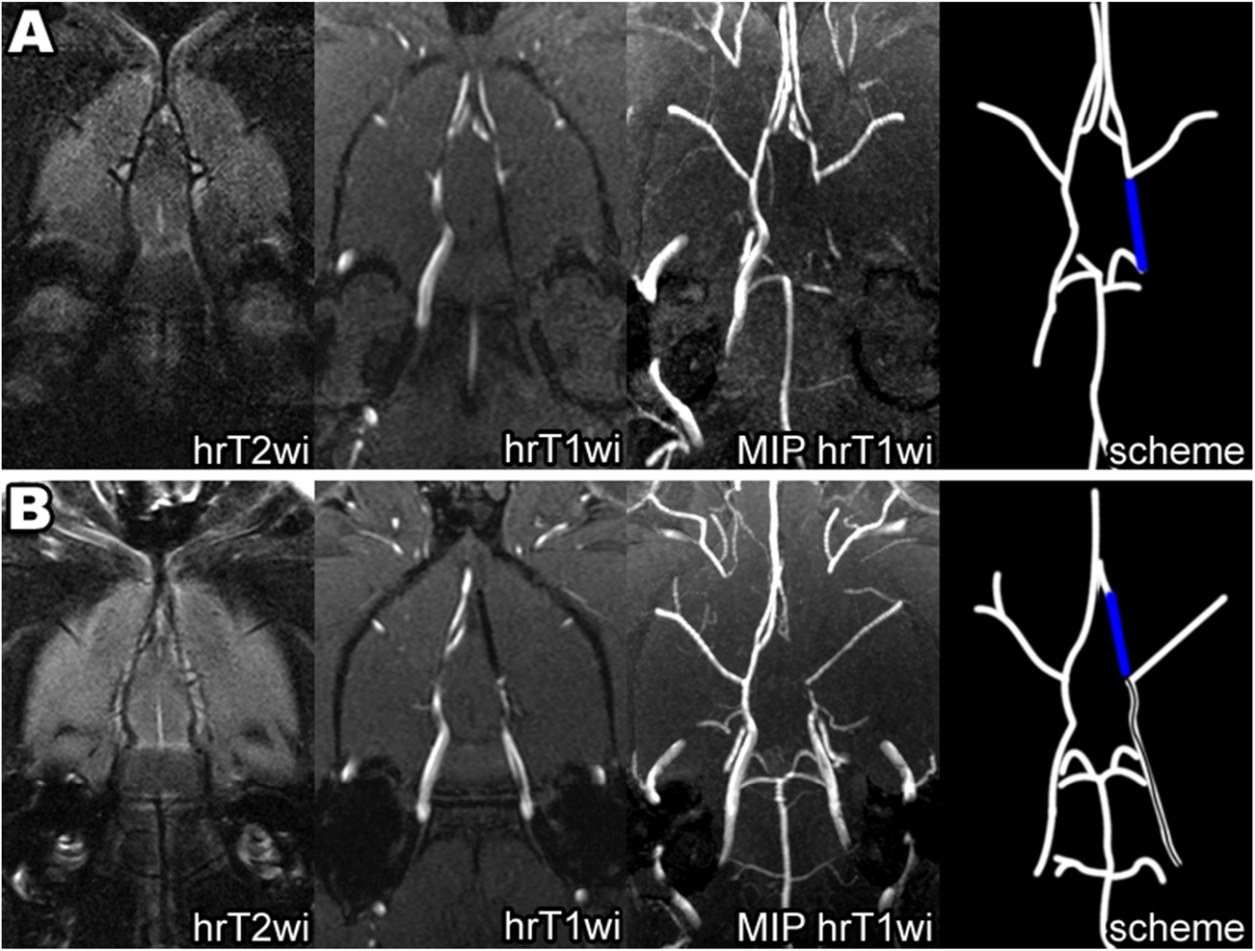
Filament visualization on MRI during MCAO: the examples of incorrect filament position with persistent blood flow in the right MCA. a The filament tip inserted insufficiently deep and visualized as elongated hypointense zone inside the distal part of ICA below the origin of MCA on hrT1wi and as the absence of blood flow in the distal part of the ICA on MIP hrT1wi (blue line on scheme). The right MCA is hypertensive on hrT1wi due to the of retrograde blood flow from ACA. b Excessively deep filament insertion into ACA is visualized as elongated hypointense zone inside the A1 segment of ACA on hrT1wi and as the absence of blood flow in the A1 segment of ACA on MIP hrT1wi (blue line on scheme). The right MCA is also hypertensive on hrT1wi due to direct blood supply from the ICA
